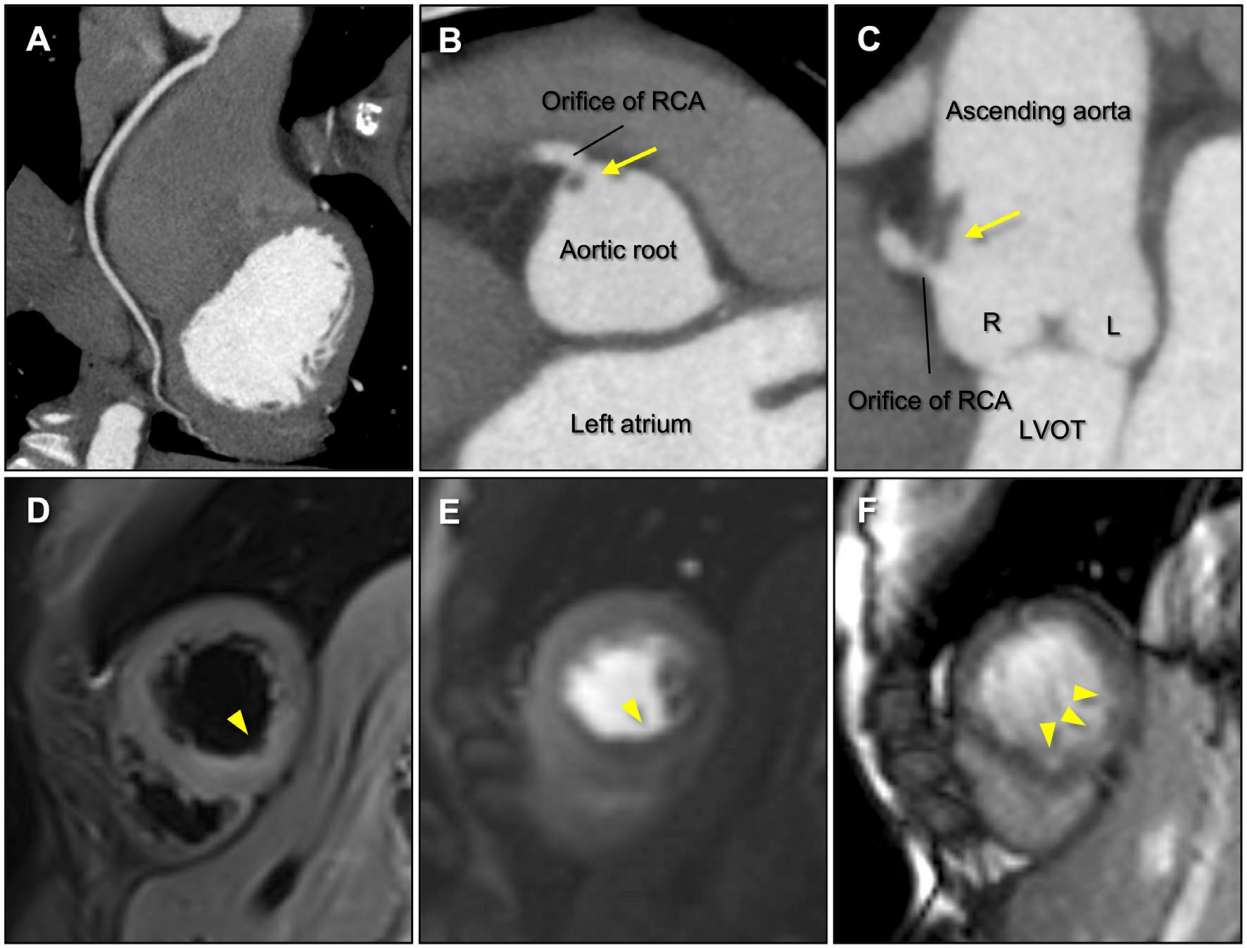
(A) On cardiac computed tomography, curved planar reconstruction image of the right coronary artery (RCA) shows no stenosis or obstruction. (B) Short‐axis image and (C) long‐axis image of the aortic root show a small thrombus near the RCA orifice (yellow arrows). (D) On cardiac magnetic resonance imaging, a high‐intensity area on the T2‐weighted short‐tau inversion recovery image indicates myocardial edema caused by acute myocardial infarction (yellow triangle). (E) Perfusion defect on the myocardial perfusion image indicates coronary flow restriction (yellow triangle). (F) Multiple subendocardial late gadolinium enhancements indicate subendocardial myocardial infarction (yellow triangles). L, left coronary aortic sinus; LVOT, left ventricular outflow tract; R, right coronary aortic sinus; RCA, right coronary artery.
